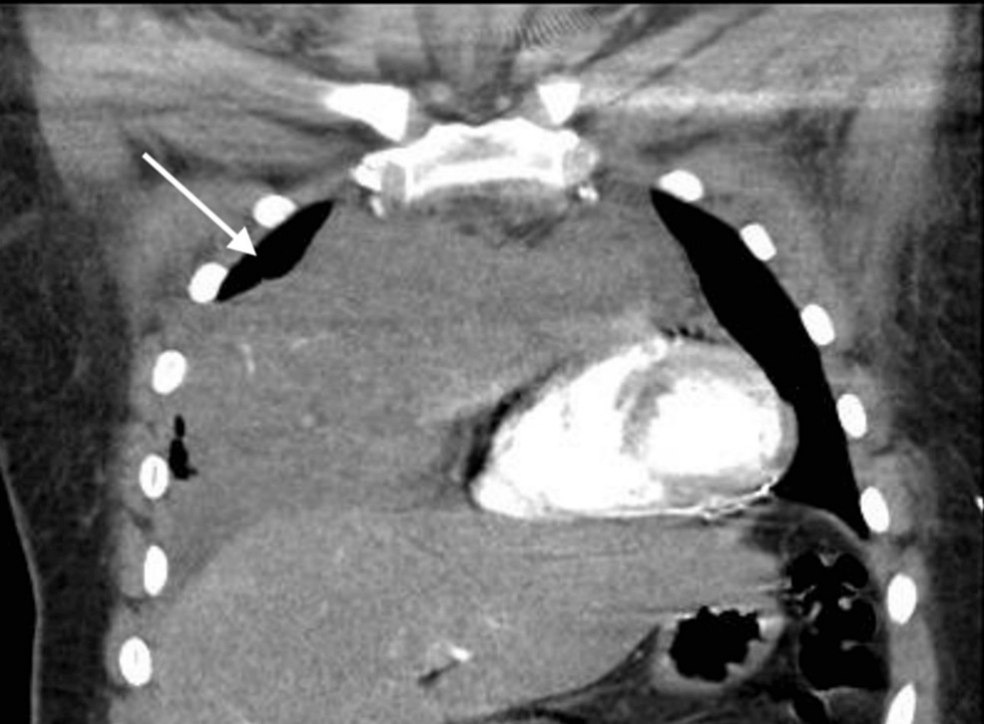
Computed tomography angiography of the chest. CT scan of the chest with coronal view showing the extent of the mediastinal mass and the mass effect on the heart. CT: computed tomography.
